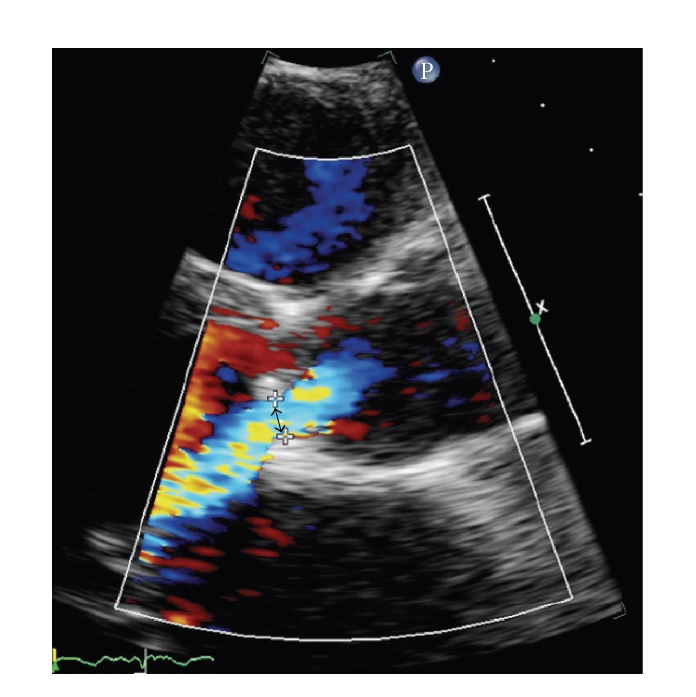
Color doppler evaluation of the aortic valve regurgitation: the arrow points to “vena contracta” of a central severe regurgitant jet.
